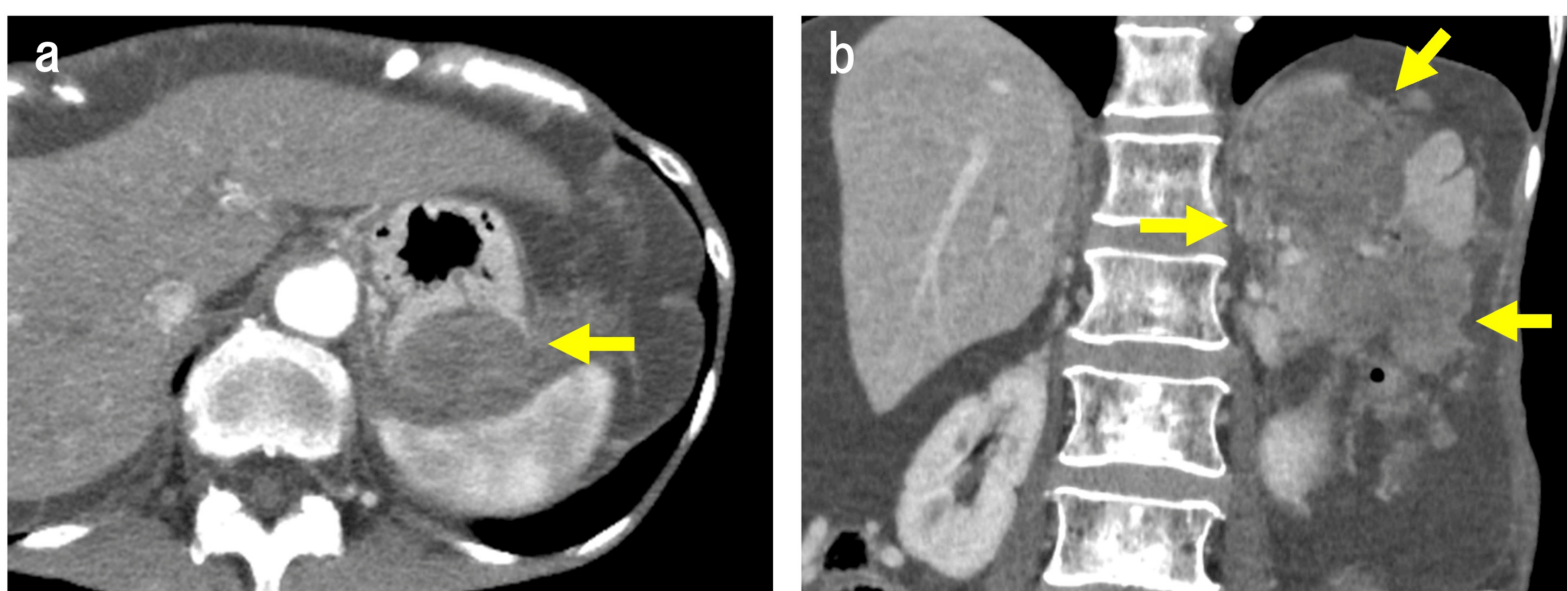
Contrast-enhanced computed tomography images (a) A 110-mm-sized exophytic gastric tumor and ascites. The gastric wall adjacent to the exophytic gastric tumor is discontinuous; however, the continuity of the gastric intraluminal surface is maintained (arrow). (b) Coronal image of exophytic gastric tumor (arrows).
